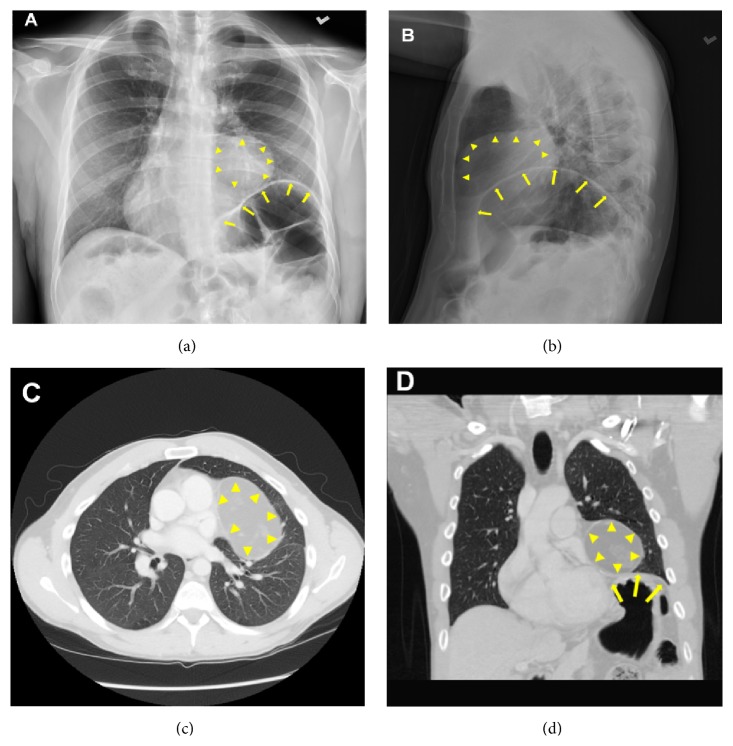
(a) Chest X-ray showing abnormal cardiac figuration with rounded left cardiac border, prominent right cardiac border, and elevated left hemidiaphragm outlines by yellow arrows; rounded mass is seen on top of the raised diaphragm (arrow heads). (b) Chest X-ray-lateral view of the mass (arrow heads) and raised diaphragm (yellow arrows). (c) CAT scan, transverse view of chest showing a well-demarcated heterogeneous, predominantly fat containing mass with a calcified outline, noted arising from the mediastinum (arrow heads), abutting the left cardiac border, and measuring 8.8 × 5.5 × 5.5 cm, with no definite invasion of the cardiac structures; and (d) coronal view of the chest prominently denoting the hemidiaphragm (yellow arrows) and mediastinal mass on top of it (arrow heads).
